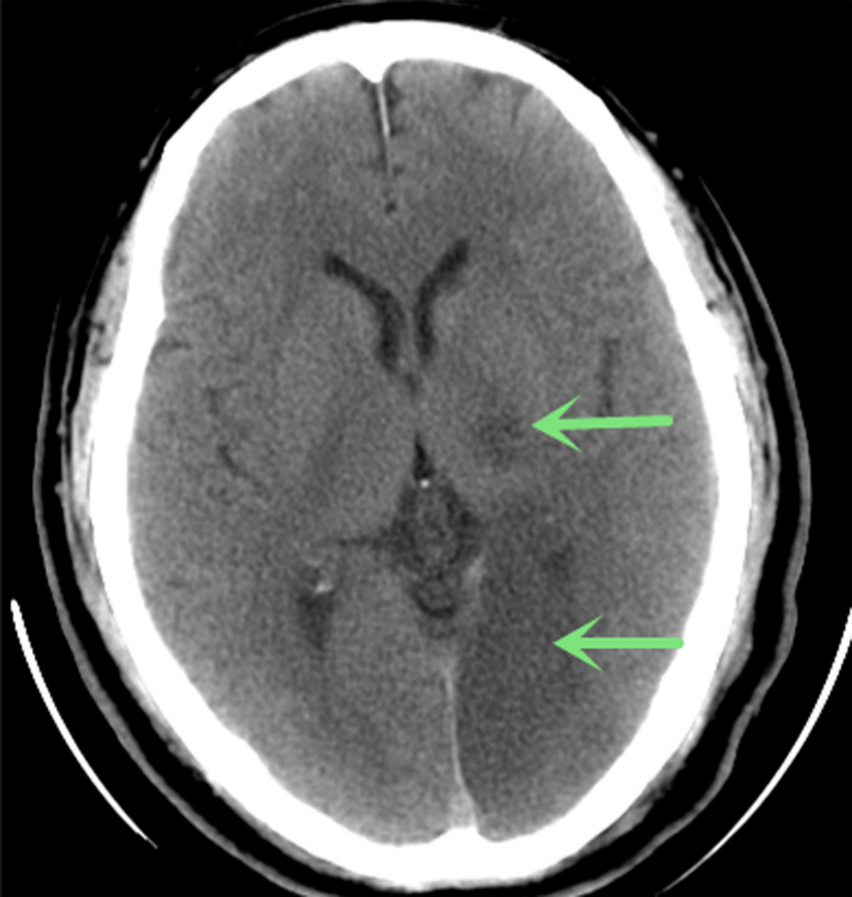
Head CT (18 h after symptom onset) showed ischemic infarcts in the left parieto-occipital lobe and thalamus (arrow).
